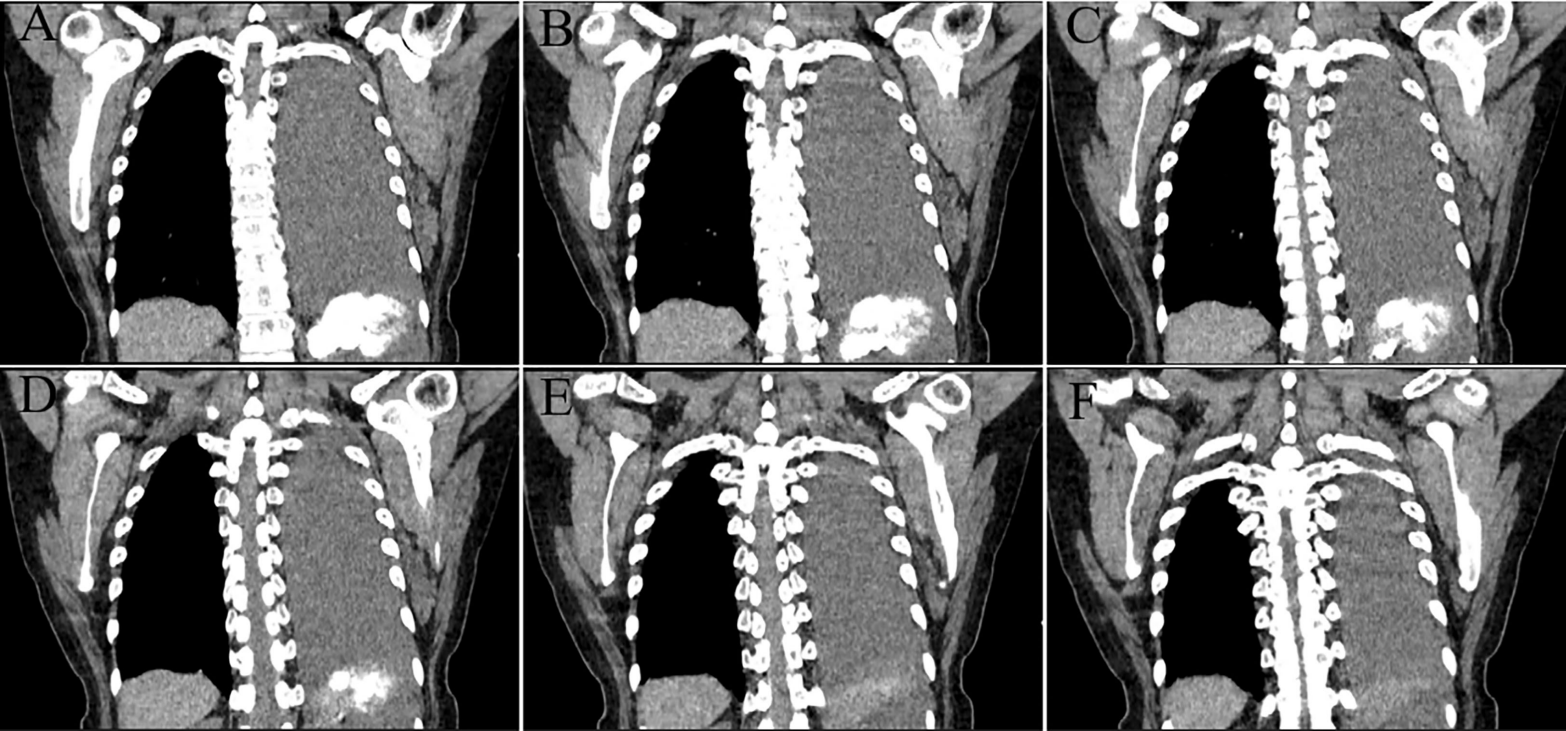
Continuous coronal computed tomography (CT) images. A mass with a high-density shadow was observed in the left thoracic cavity, with an irregular boundary and adjacent pleural thickening and adhesion. We intercepted a section of the continuous CT images from the appearance of the spinal canal to the disappearance of the tumor (A–F), all of which showed no involvement of the spinal canal by the tumor.
